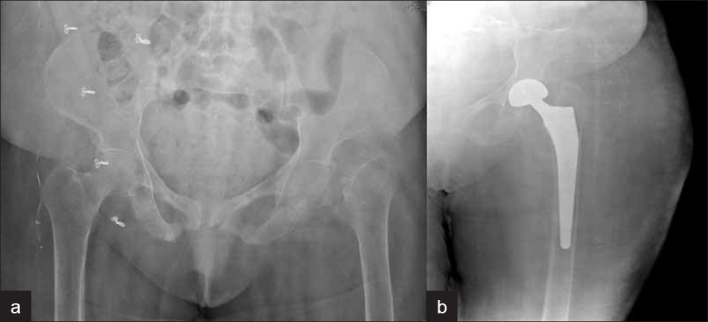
Radiographs (anteroposterior view) of 83-year-old patient with fracture neck of femur on left side. (a) Preoperative; (b) 24 months after cementless bipolar hemi-replacement
